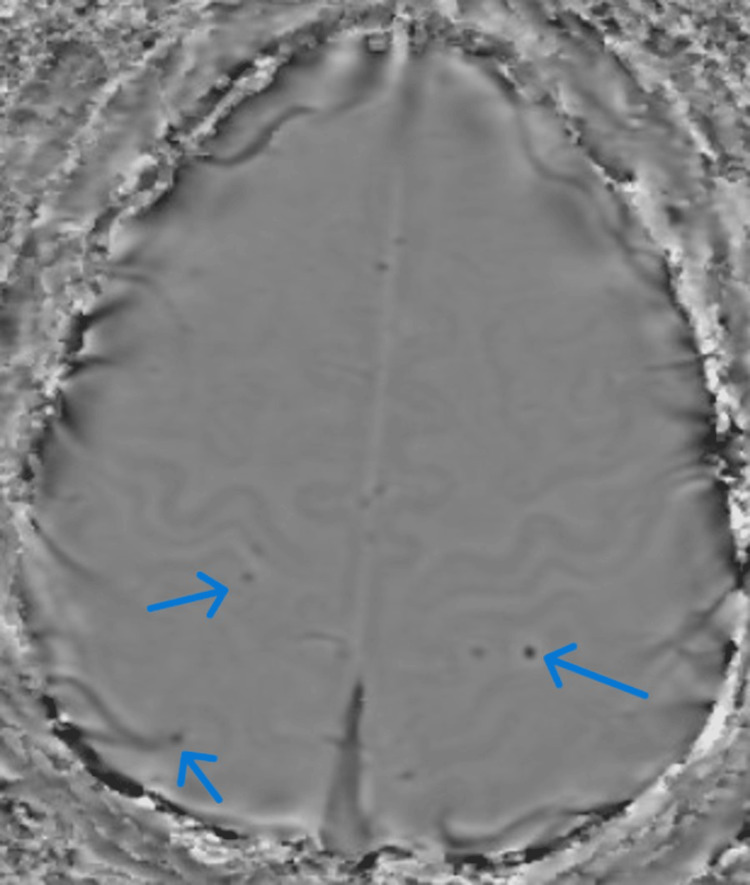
Contrast-enhanced MRI brain film showing blooming at parieto-temporal areas suggestive of microbleeds
